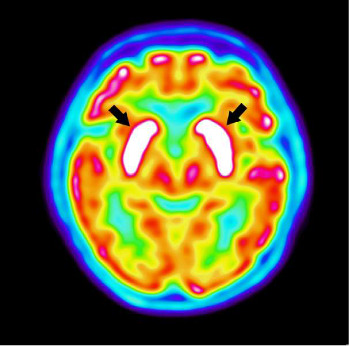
Fluorodeoxyglucose positron emission tomography (FDG-PET) with an intense and diffuse bilateral hypermetabolism in the striatum (black arrows).
